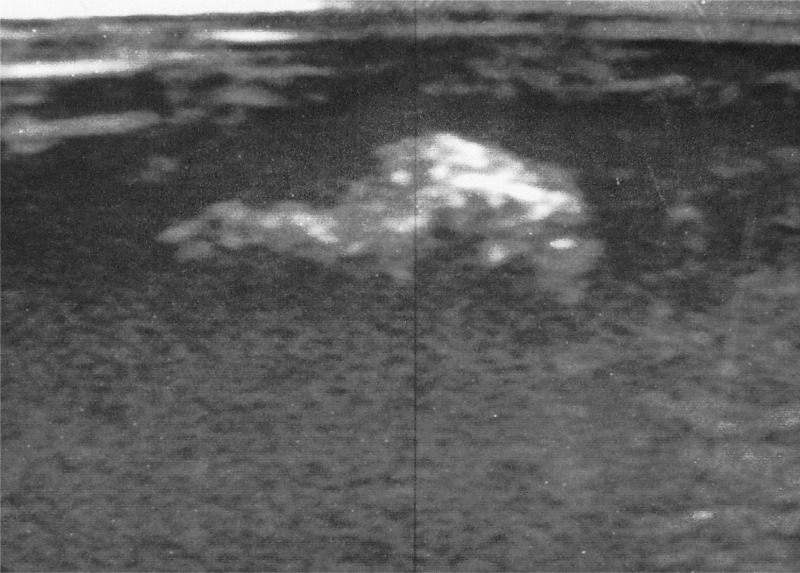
Ultrasound showed fusiform echogenic image.
